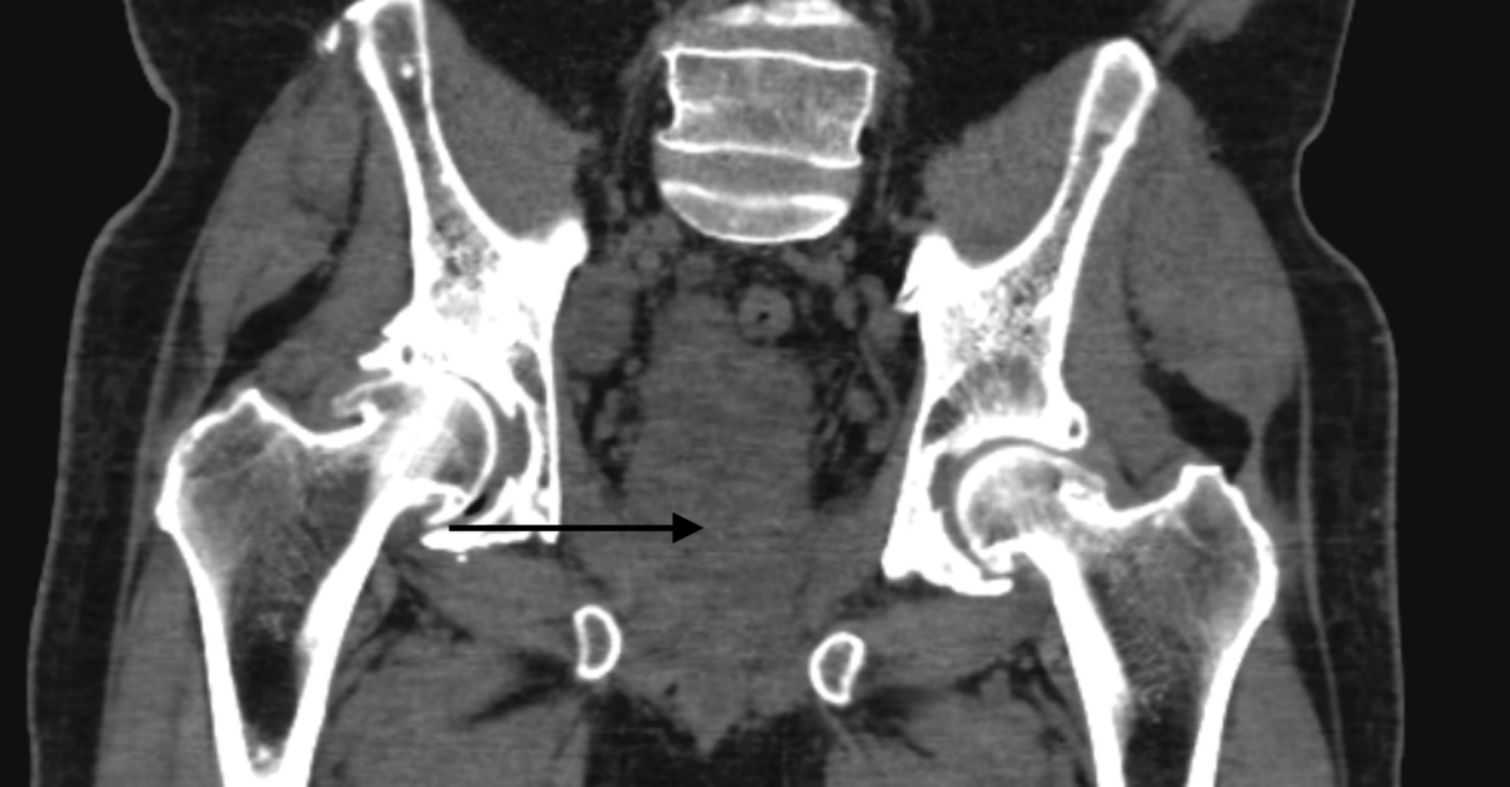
Coronal view of the CT pelvis Black arrow points toward the prostate
